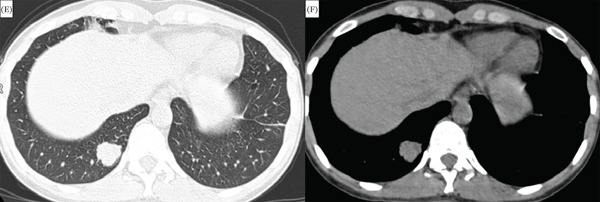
Nodular amyloidosis. (E) Axial chest CT (lung window) displays a solid parenchymal nodule with irregular margins in the right lower lobe. (F) Mediastinal window of the same lesion.
